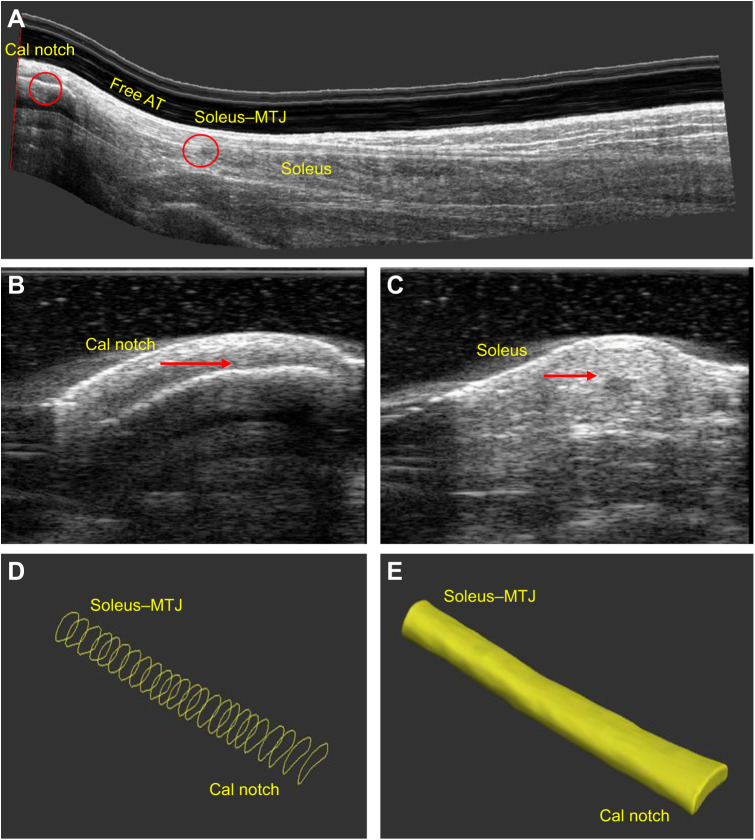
3D Achilles tendon image analysis. (A) Sagittal plane of a 3D ultrasound image of the free Achilles tendon (AT). Two anatomic landmarks [calcaneal notch and soleus–muscle–tendon junction (MTJ)] were identified on the (A) sagittal plane and (B,C) transverse plane for calculation of AT length. Cal notch, calcaneal notch. (D) Manual contouring of free AT cross-sections from calcaneal notch to soleus–MTJ. (E) Free AT volume reconstruction.
